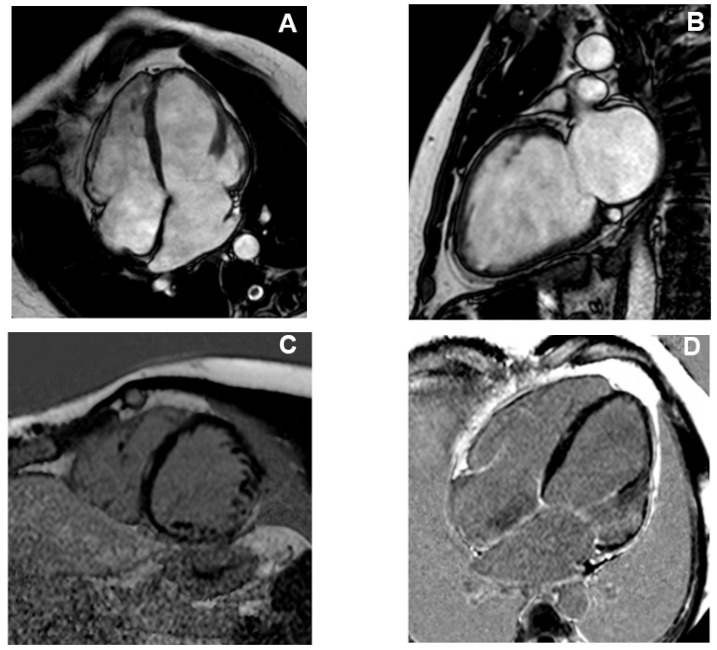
A patient with DCM and severe LV impairment (EF 25%) with a dilated left ventricle, as shown in balanced steady-state free precession imaging (bSSFP) in 4-chamber view (A) and 2-chamber view (B). LGE imaging in the short axis (C) and vertical long axis (D) shows the presence of LGE in the interventricular septum and the inferior and lateral walls. The patient developed an episode of VT, which was effectively terminated by the ICD (implanted following MRI).
